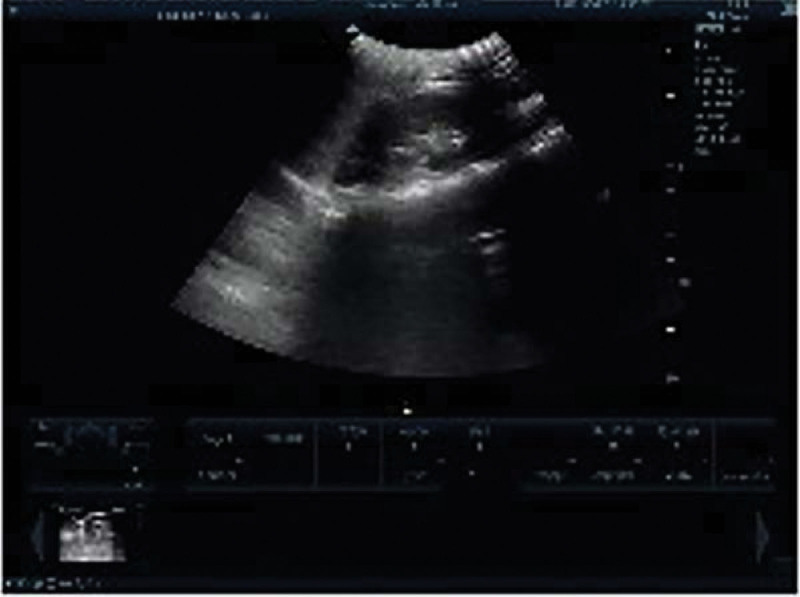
Postoperative ultrasonography (Case 1).
